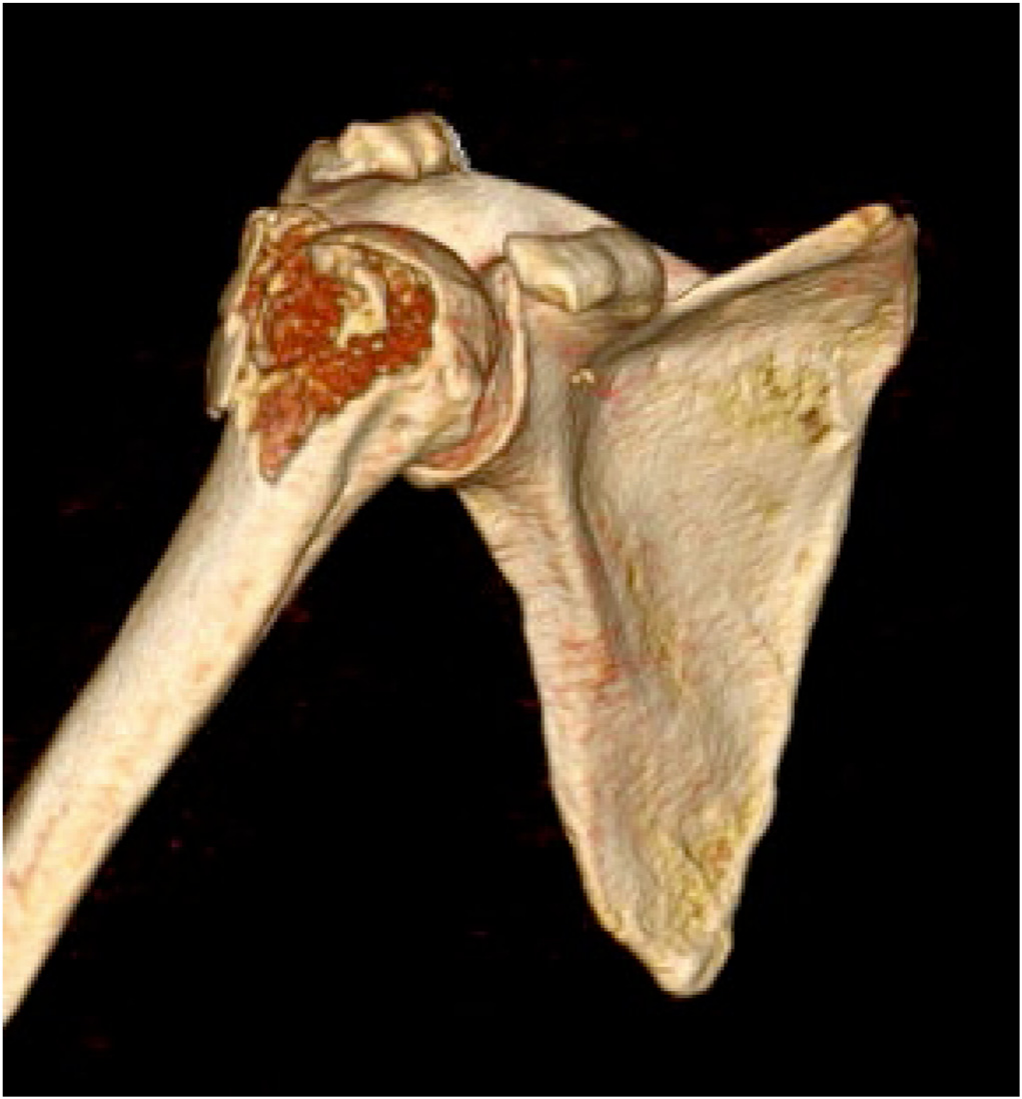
This is a CT-based 3D surface model reconstruction of a right shoulder demonstrating a comminuted fracture of the greater tuberosity that is displaced posteriorly and superiorly. Note the extension of the fracture lateral to the level of the surgical neck of the humerus. *CT*, computed tomography; *3D*, three-dimensional.
